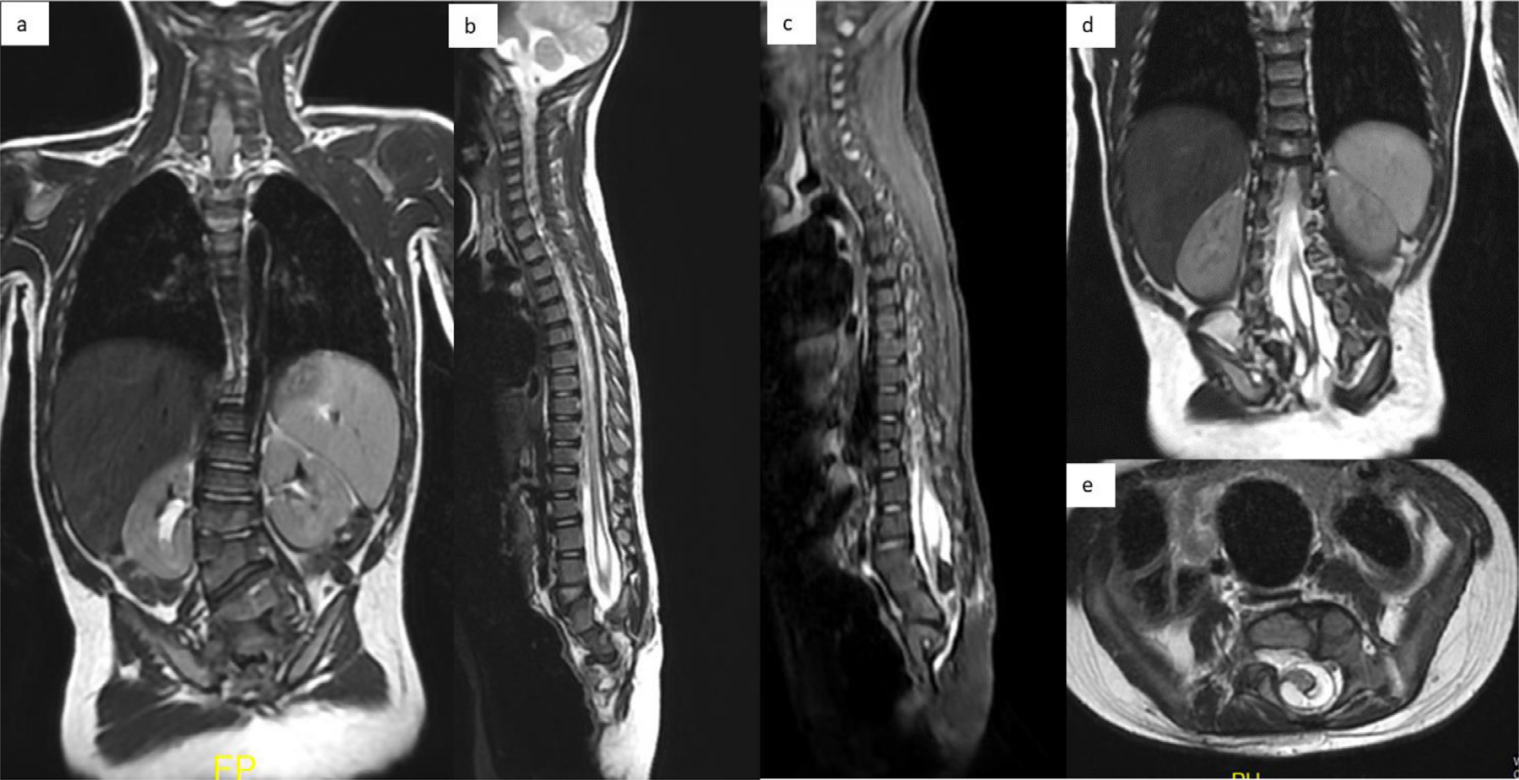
(A-E) An MRI of the entire spine using T1-T2 sequences reveals a failure of lumbar segmentation or the presence of hemivertebrae (A) and partial sacral agenesis (B and C) consistent with Pang type IV. This malformation is indicated by the low-lying conus and sign of syringomyelia (B-D). In this case, the retained medullary cord with the conus located below the lumbar was found to be attached to fatty structure (D and E).
